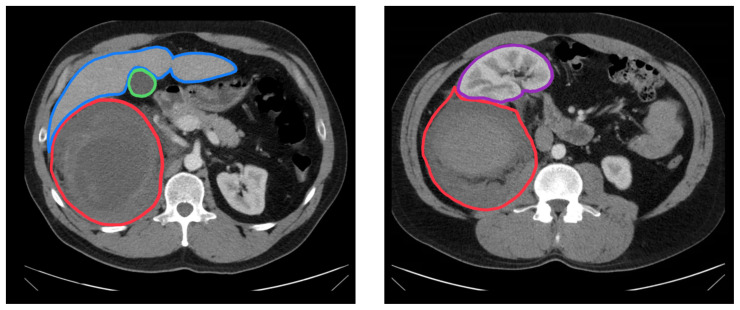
Unannotated CT Video Link: https://youtu.be/fKWMmcR8T4M Annotated CT Video Link: https://youtu.be/bZGjvnpo1B0
